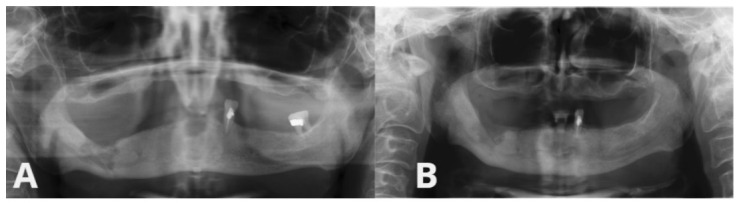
Radiological assessment of Patient 4. (A) Preoperative orthopantomogram (OPG) showing MRONJ lesion. (B) OPG 6 months after surgical intervention and PRF application, showing radiological improvement.
